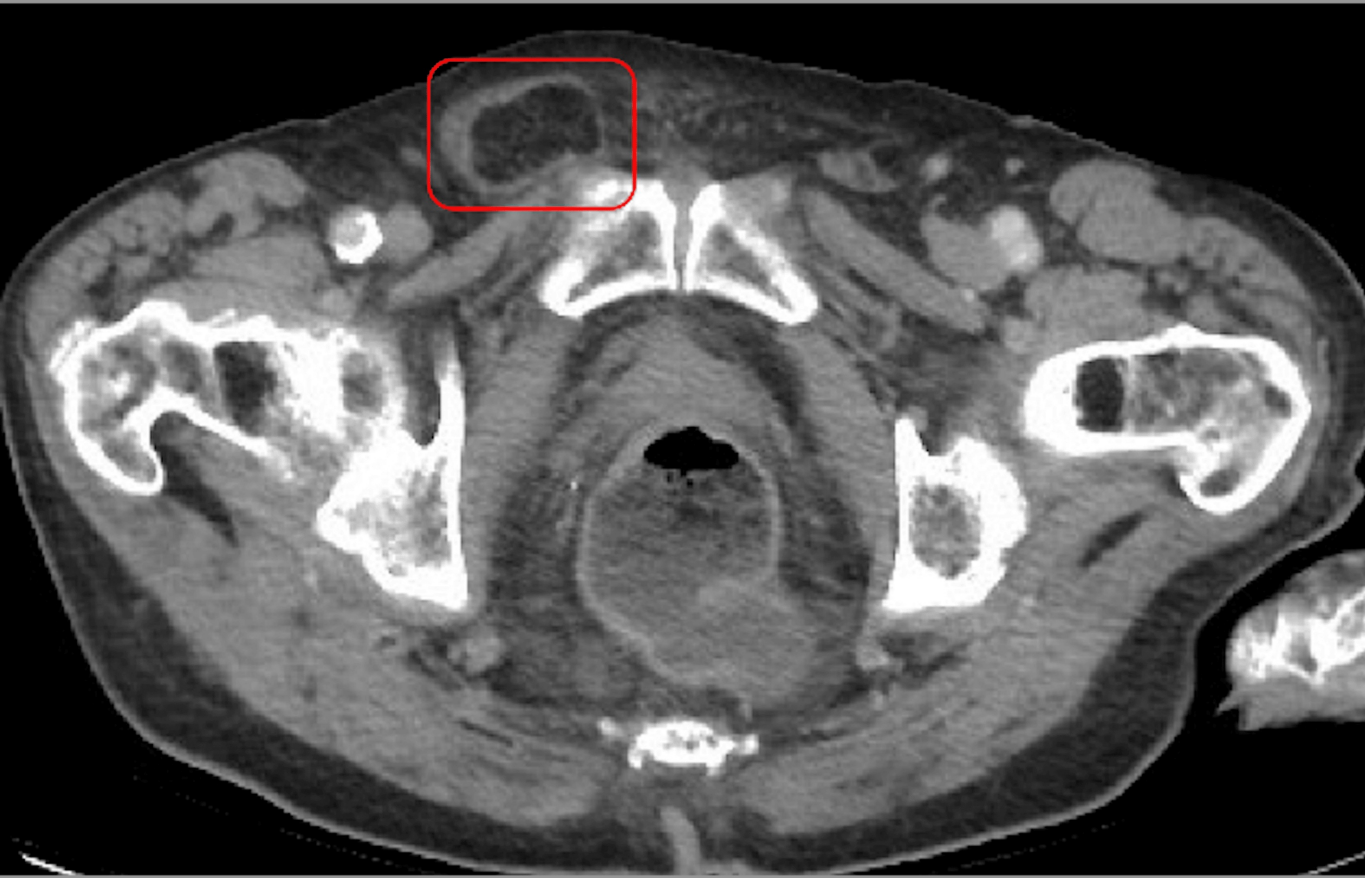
CT of the abdomen and pelvis without contrast (axial view) showing postsurgical changes noted within the left groin related to a previous inguinal hernia repair and a right inguinal hernia identified containing fat and a small volume of fluid (red box).
